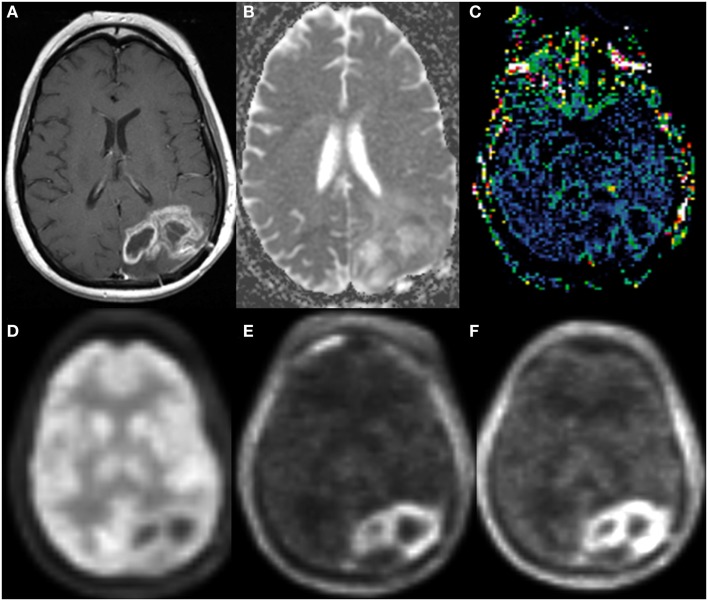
Enlarging left parieto-occipital lobe enhancement 4 months following chemoradiation therapy (A). The enhancing region demonstrated low ADC (B) on DWI, mildly elevated rCBV (C) on DSC-MRI. FDG-PET (D) of the same lesion had less conspicuous lesion to background uptake compared to FLT-PET (E) and FET-PET (F). Subsequent resection confirmed progressive/recurrent glioblastoma.
